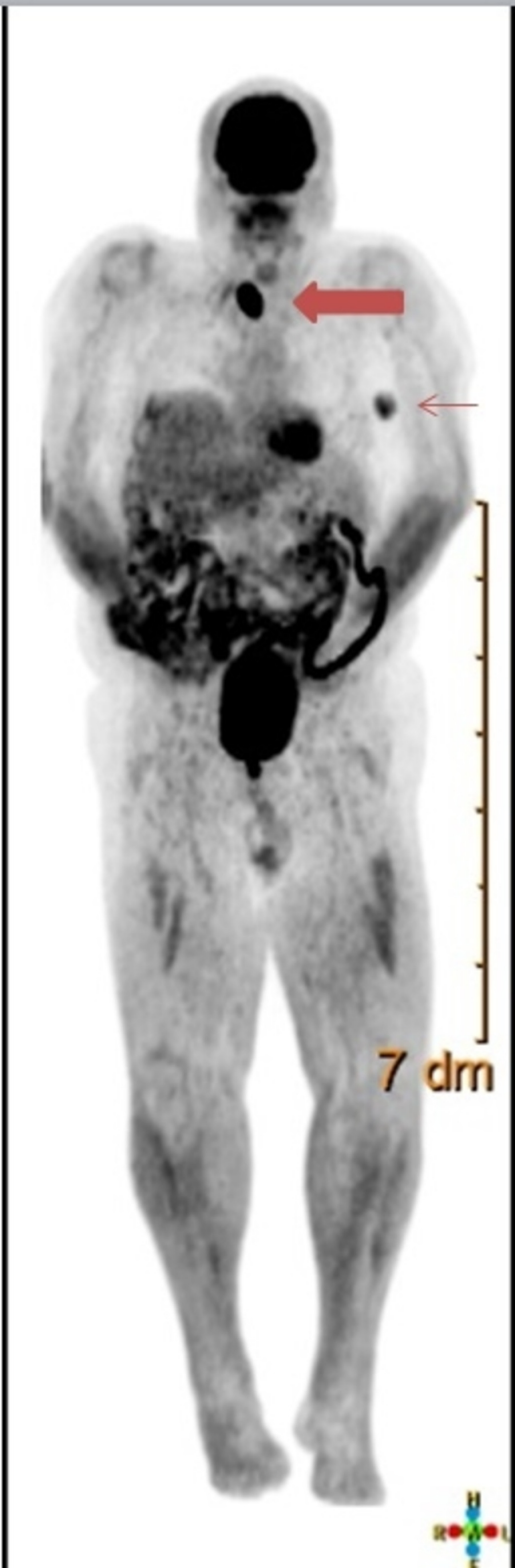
PET/CT scan The right thyroid gland (thicker arrow) is enlarged with increased metabolic activity with a maximum SUV of 14.4, which is suspicious for malignancy. The left axillary lymph node (thinner arrow) is enlarged with a maximum SUV of 4.3, which is concerning for melanoma involvement.
